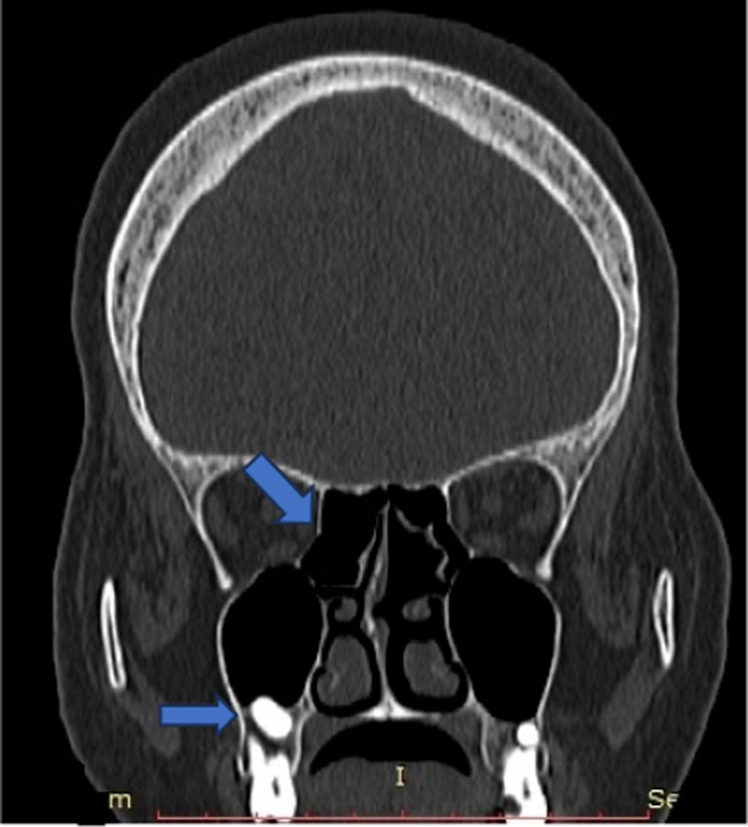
CT coronal image shows extension of maxillary sinus to teeth roots, in ethmoid sinus ethoidal bulla is seen.
